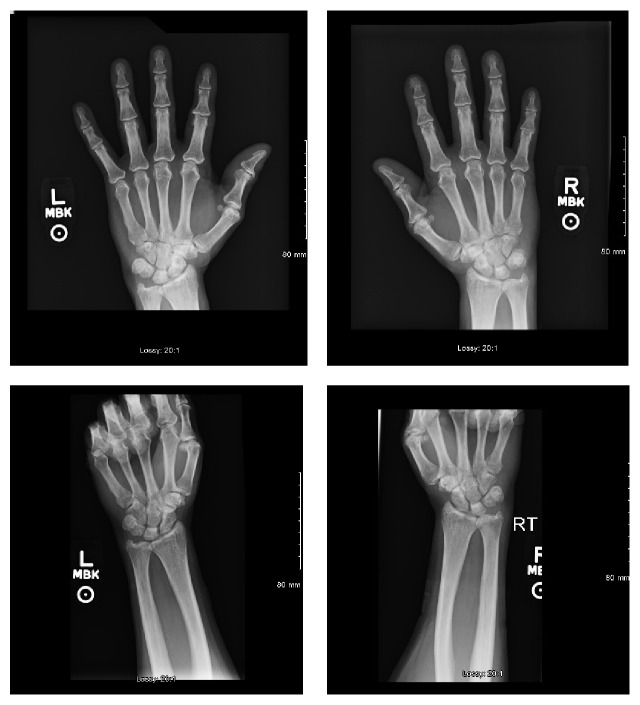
X-ray of the bilateral hands showing early inflammatory arthropathy.
